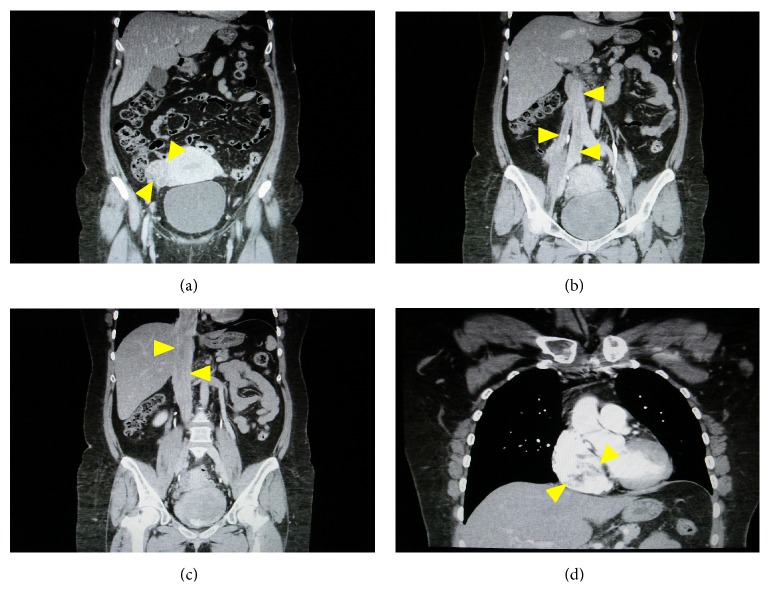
Computed tomogram of the chest, abdomen, and pelvis showed a large mass within the inferior vena cava, extending into the right atrium and ventricle. (a) Coronal image demonstrates large filling defect in the right parametrium. (b, c) Coronal image presents large filling defect in the inferior vena cava extending from both the right uterine vein and common iliac and the right ovarian vein to the inferior vena cava and the right atrium. (d) Coronal image demonstrates large filling defect in the right atrium.
